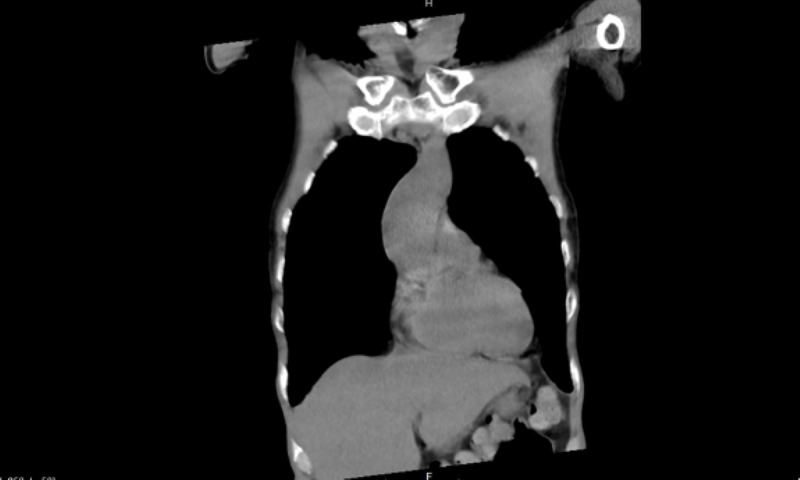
CT chest without contrast revealed fluid-filled dilated esophagus, which was suboptimally evaluated due to lack of contrast. The patient also had left lower lobe consolidation consistent with pneumonia.
